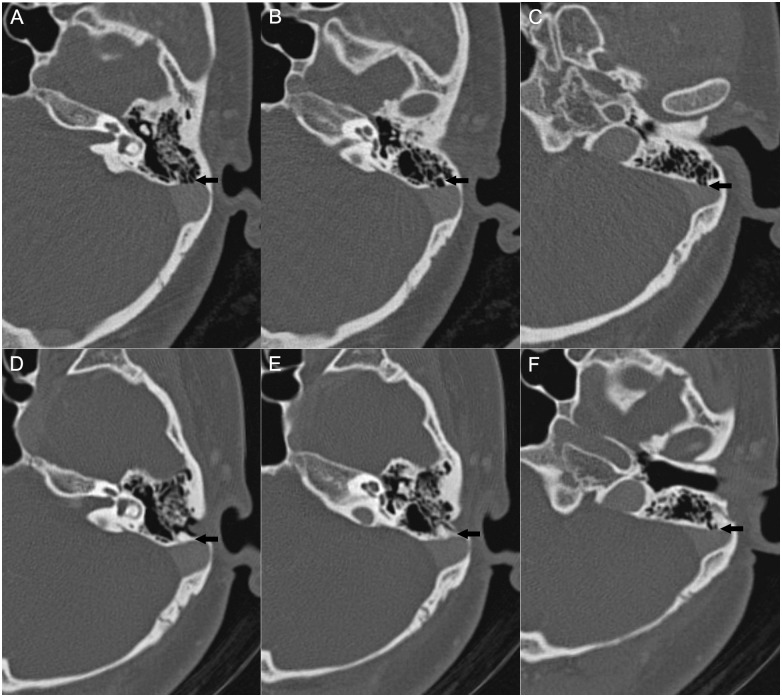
Preoperative (A–C) and postoperative (D–F) CTA images of a patient (Patient 9) with partial resolution of PT following surgery. The layers in (A), (B) and (C) correspond to those in (D), (E) and (F), respectively. The dehiscences in layers (A) and (B) are repaired postoperatively as shown in (D) and (E); whereas the dehiscence in layer (C) is not repaired as shown in (F).
